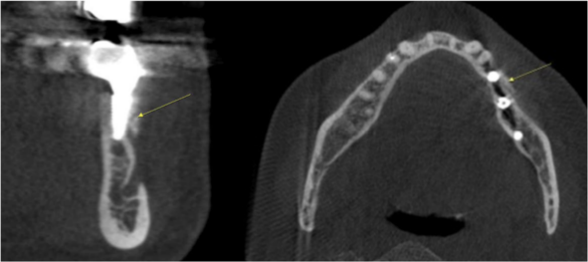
CBCT, 2 years after the restoration. The alveolar bone where bone graft using the tunneling technique is marked by a *yellow arrow*
